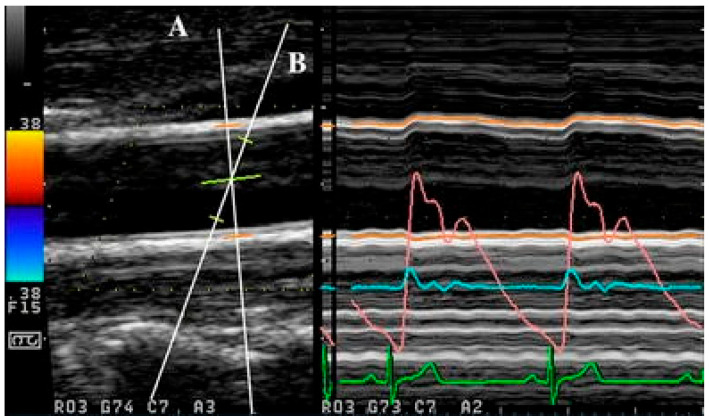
Simultaneous acquisition with two ultrasound beams (A and B), with overlap at the range gate region (from [156] with permission).
